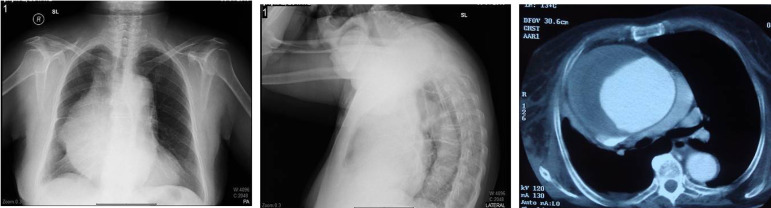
(A and B) – Aneurysm appearance on posteroanterior and lateral chest radiography. (C) – Aneurysm appearance on computed tomography scan.
